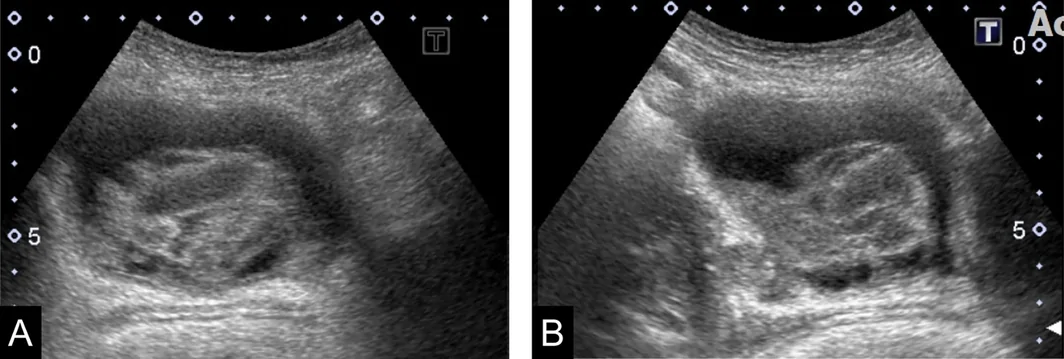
Ultrasonographic findings demonstrating a large intravesical hematoma occupying most of the bladder lumen. (A) Transverse view. (B) Sagittal view.
